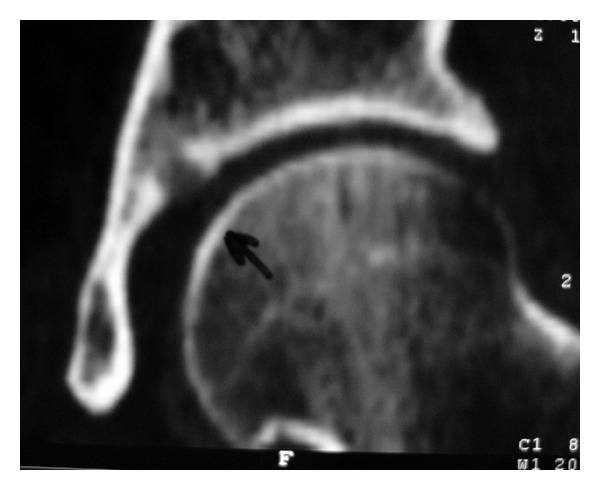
Coronal CT scan showing the lesion at the junction of the dome and notch of the acetabulum.
